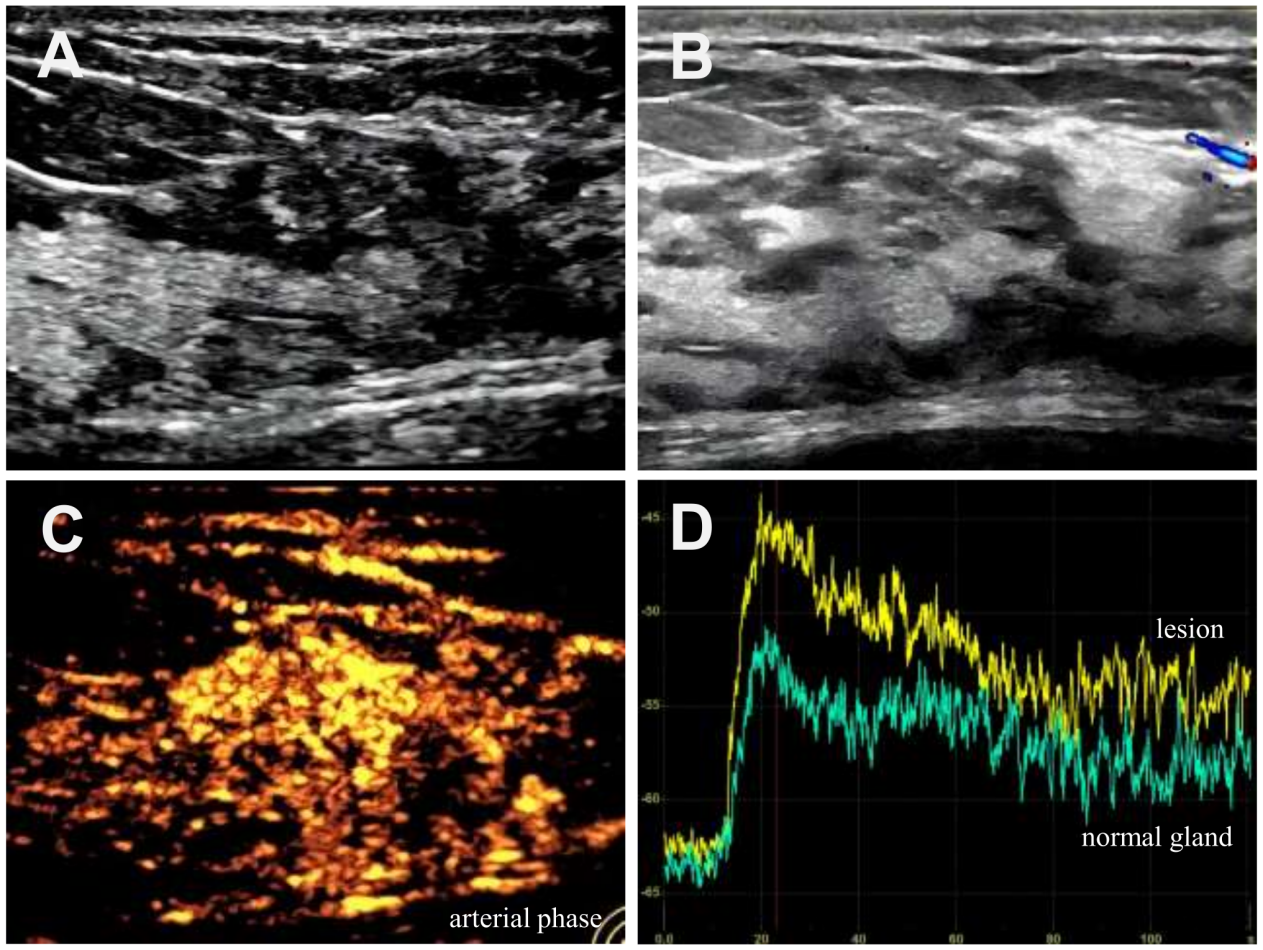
Patient underwent multimodal ultrasound examination after chemotherapy. (A) Gray-scale ultrasonography revealed a structurally disordered area, measuring approximately 26×11 mm, at the 3 - o’clock position of the left breast. This area had an irregular shape, inhomogeneous internal echogenicity, and well-defined margins. (B) CDFI detected no evident blood flow signals either around or within the nodule. (C) On arterial-phase CEUS, the lesion developed a well-defined margin after contrast enhancement. Contrast agent within the lesion was heterogeneous, and no penetrating vessels were detected in the perilesional area. (D) Quantitative CEUS time-intensity curve. The yellow and green curves represent the lesion and the surrounding glandular tissue, respectively.
